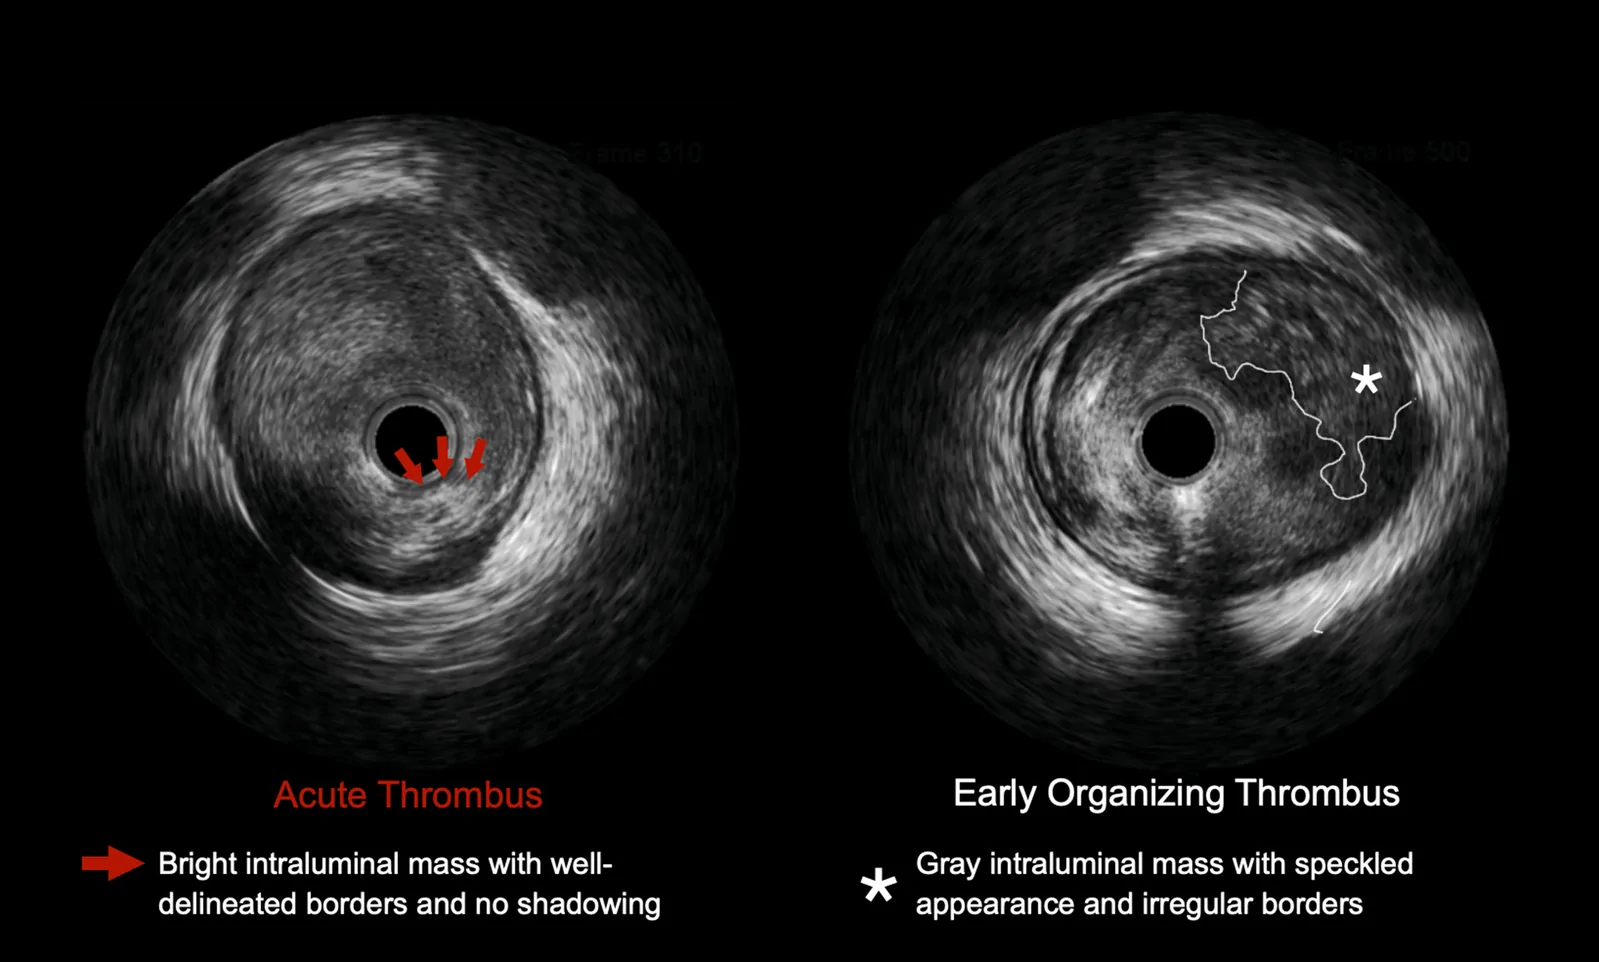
Intravascular Ultrasound Demonstrating Well-Organized Thrombus in Case 2 Intravascular ultrasound imaging of the right coronary artery demonstrating near-circumferential organized thrombus appearing as a dark, homogeneous intraluminal mass with smooth borders characteristic of densely organized, fibrin-rich thrombus.
